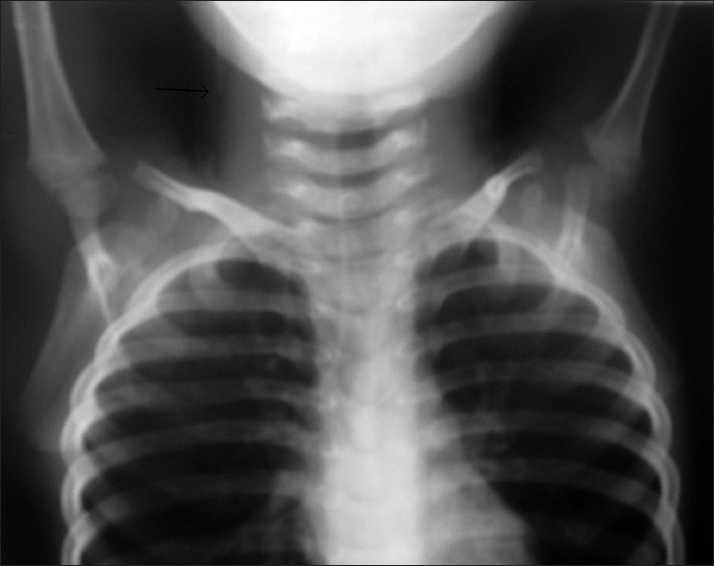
Chest X-ray of the patient shows no catheter in this view and cut of the catheter at the neck
